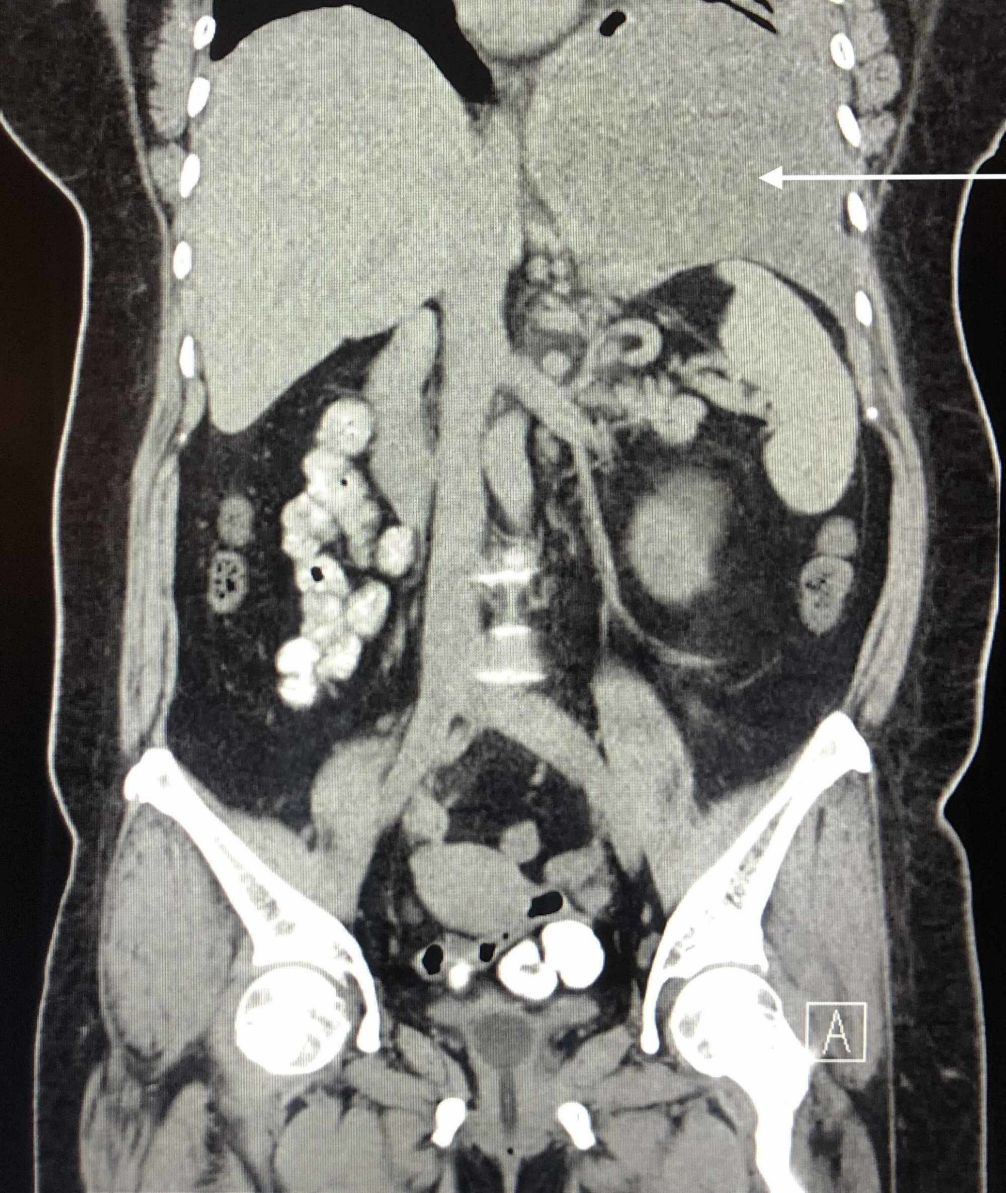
Abdomen and pelvis CT shows left lung mass isolated superior to the diaphragm.
